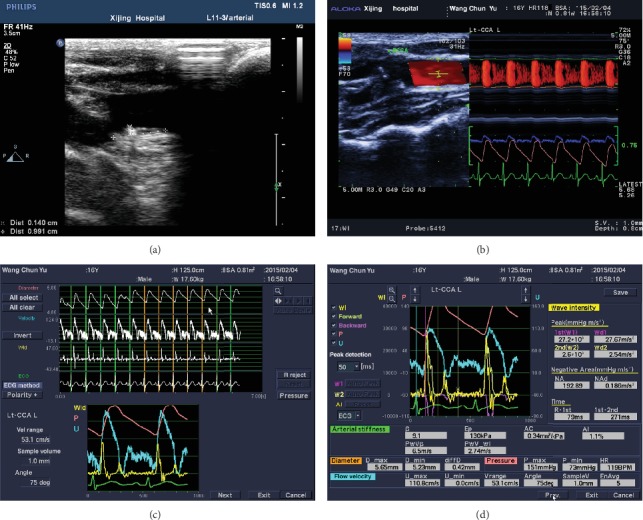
The carotid artery ultrasound evaluation of HGPS patients. (a) The borders of the plaque in the carotid artery in HGPS patients. (b) Left: screenshot of the RCCA tracing gate. Right: changes in vessel diameter. The Doppler flow and flow velocity were determined by ALOKA e-tracking. (c, d) B-mode, indicating the cursor locations and postanalysis screen.
